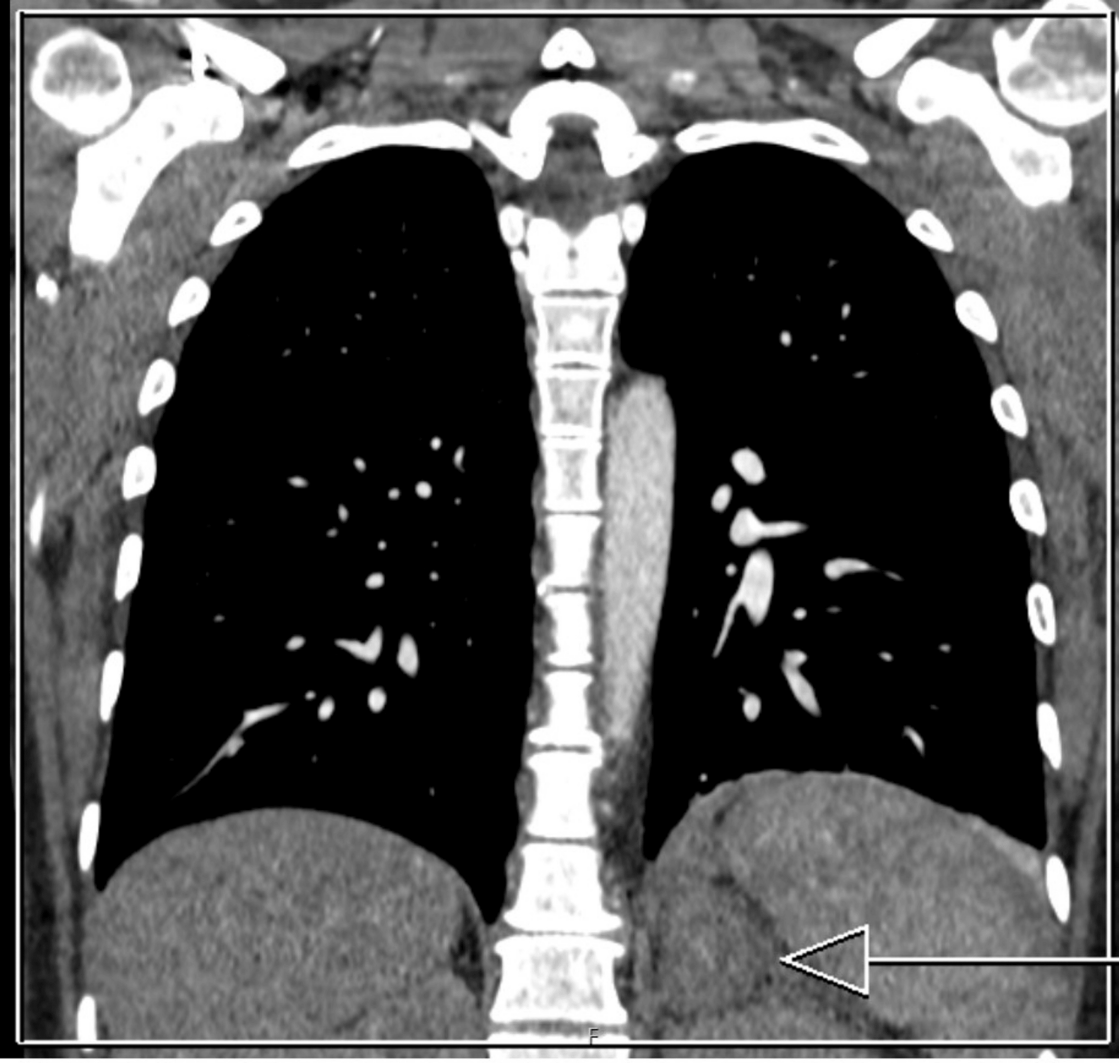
Coronal CT pulmonary angiogram showing an enlarged left adrenal gland (arrow).
